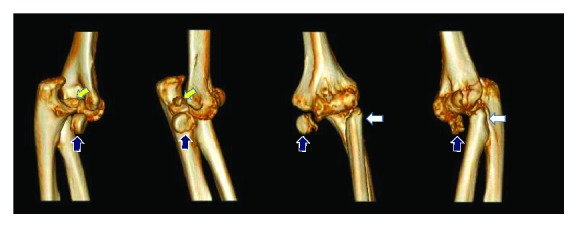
CT scan showing the coronoid tip fracture (yellow arrow) and irreparable radial head fracture with indented articular surface (blue arrow) and deformation of the fracture ends (white arrow).
